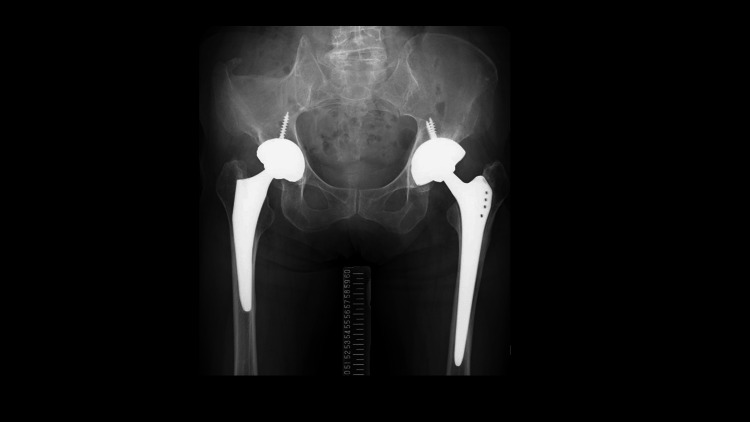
Hip radiograph obtained during the last follow-up (one year after the two-stage revision surgery)
